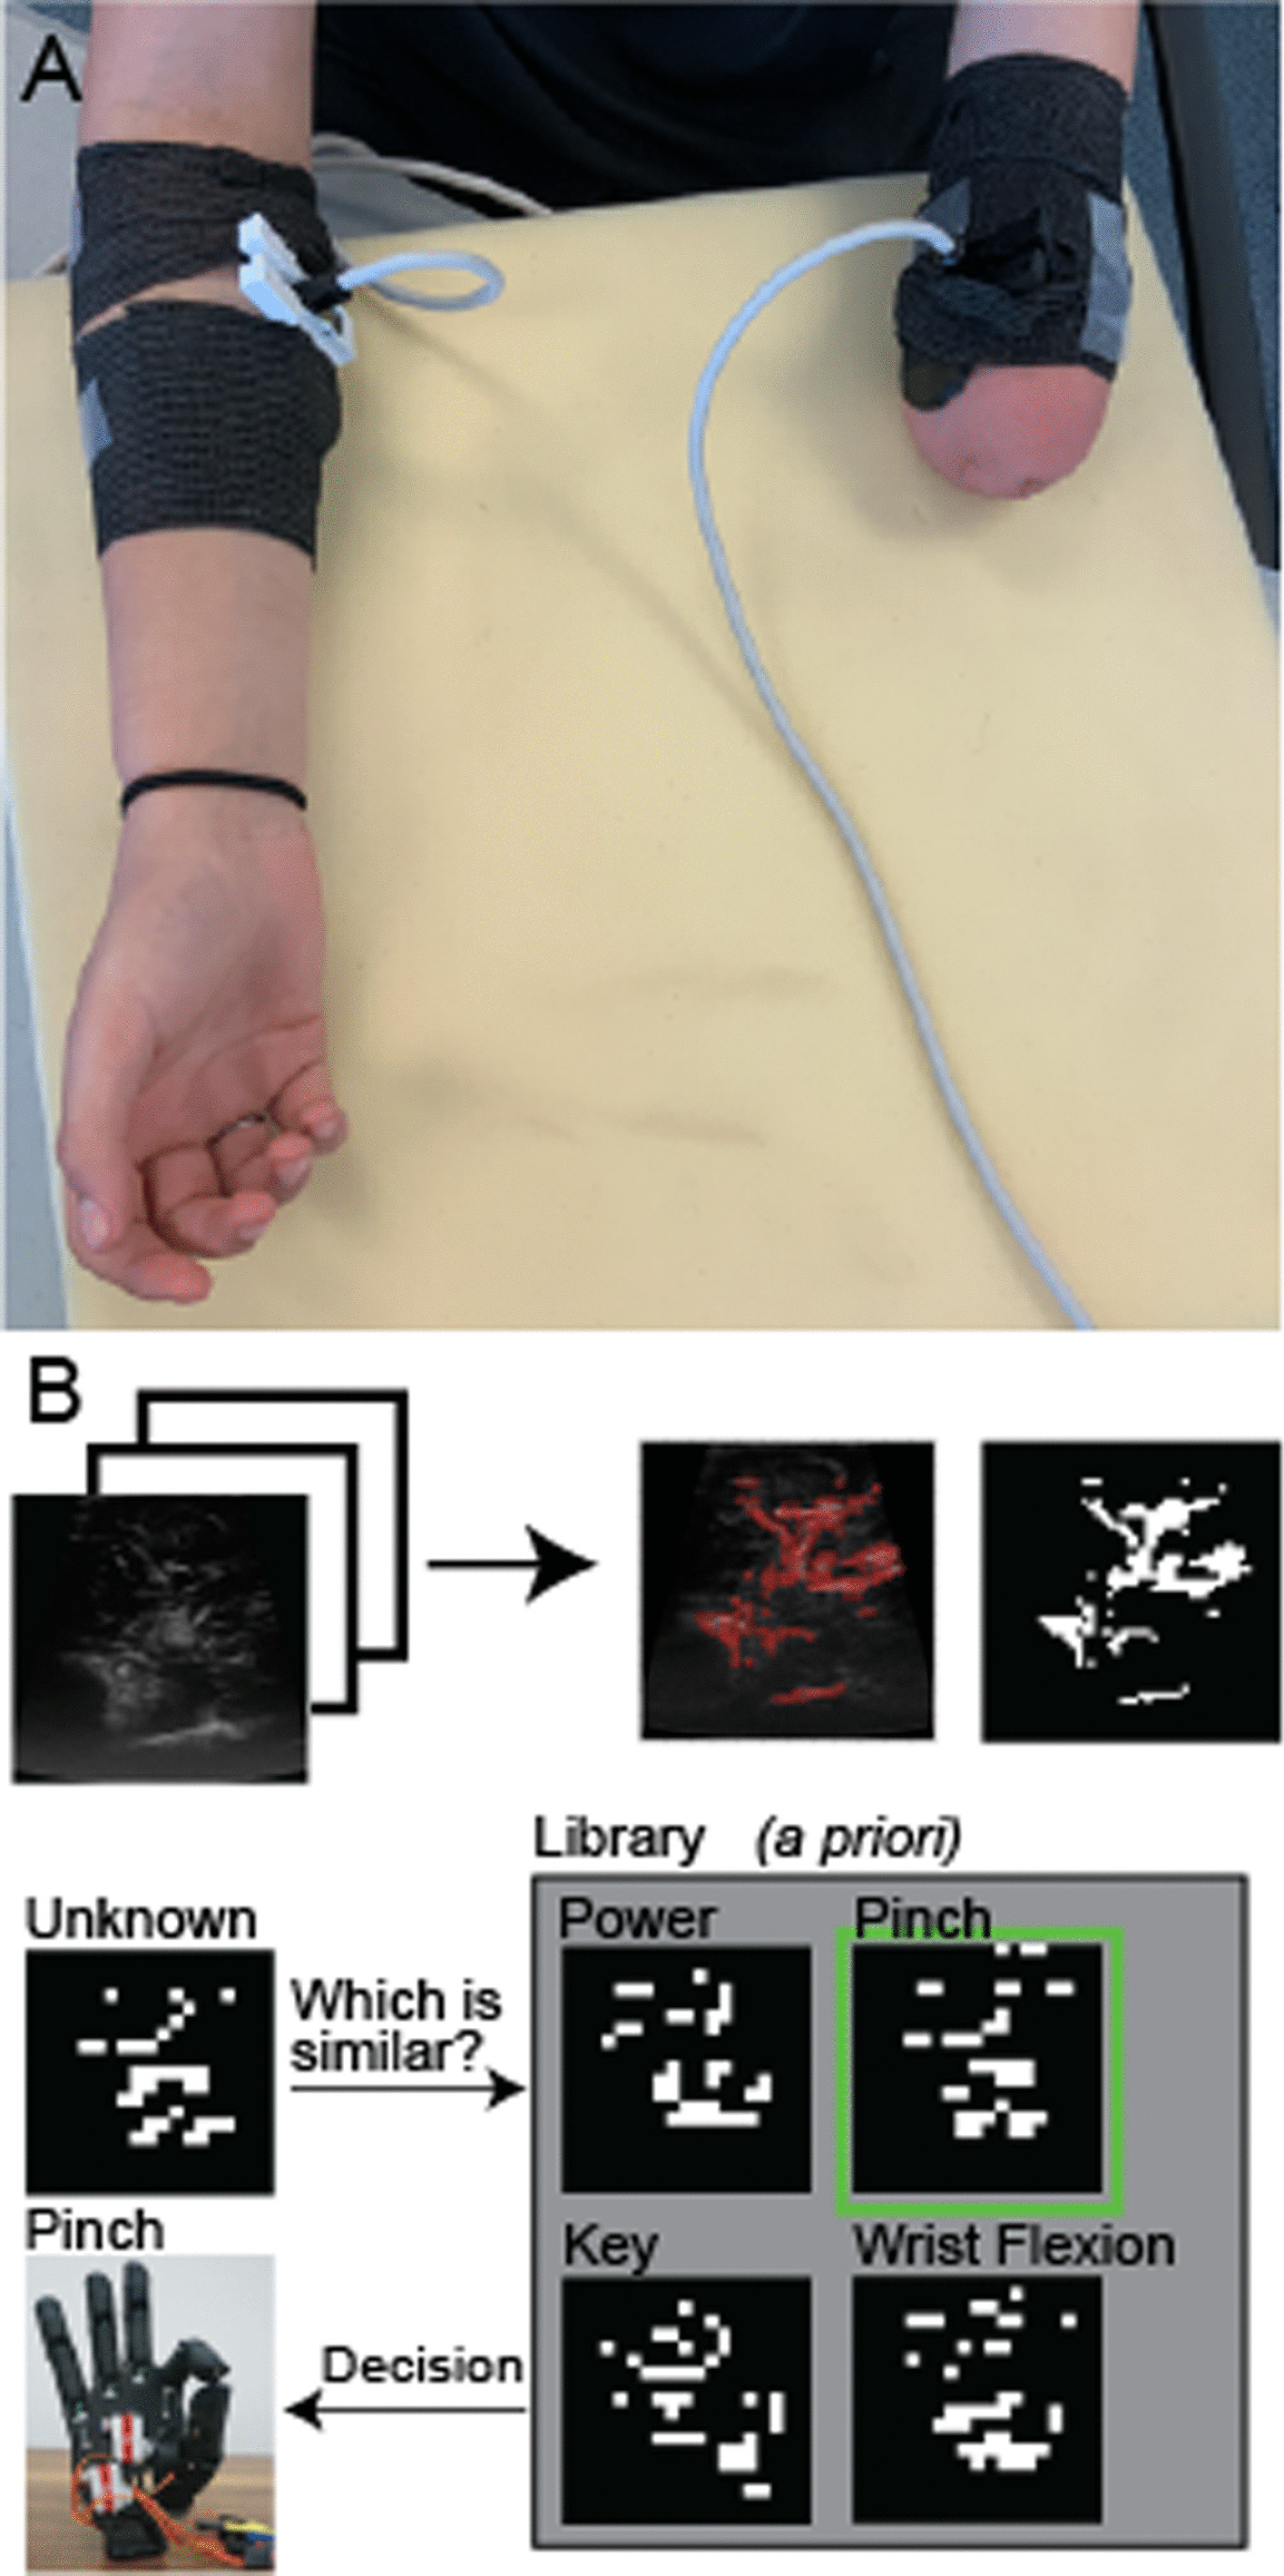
Representative placement of ultrasound transducer and visualization of machine learning algorithm. A Ultrasound transducers were attached to each limb of the participant with a 3D printed cuff and secured with self-adhesive Coban wrap. Ultrasound data was streamed directly from the Terason ultrasound system to a secondary computer for storage and offline analysis. B Spatiotemporal tissue deformations of each tested motion were filtered and scaled to create a feature set for the KNN algorithm. This supervised algorithm uses the known labels of the nearest known samples to classify an unknown sample
